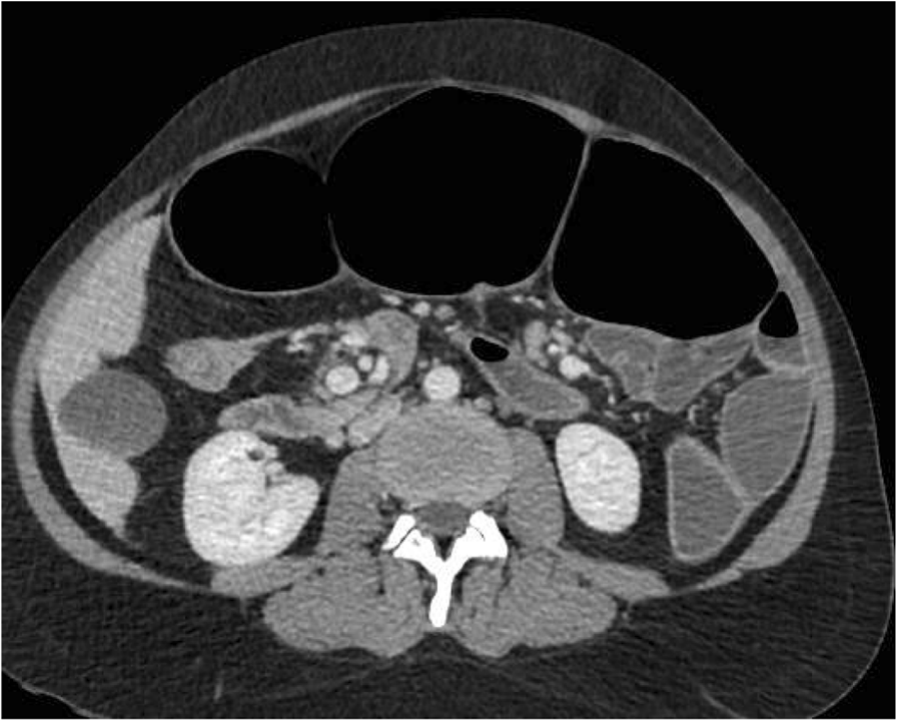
Abdominal axial cross-sectional computed tomography showing hydroaeric small intestine distention with pneumatosis of the small intestine loops
